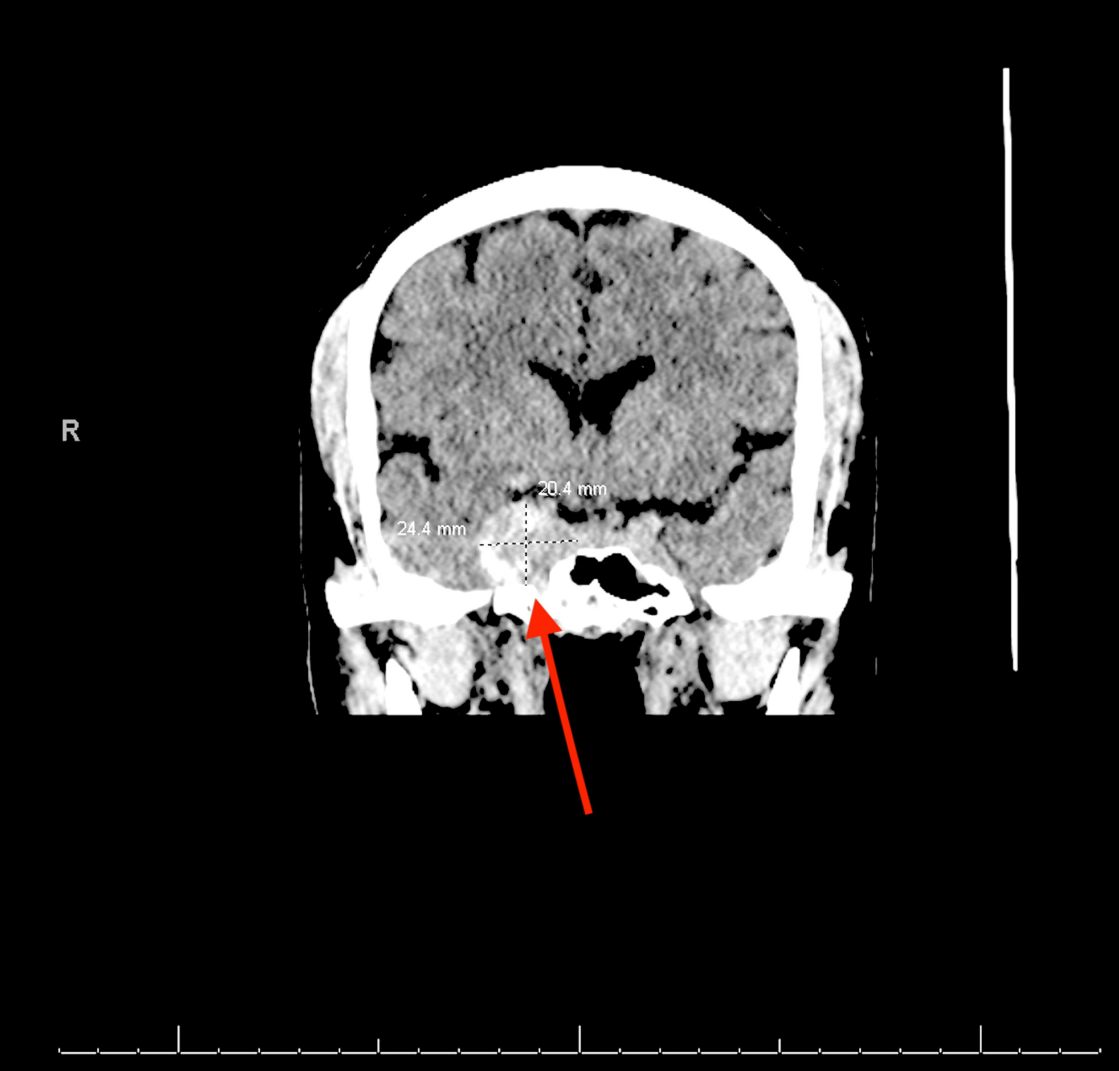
Original CT head from the emergency department The arrow points to the aneurysm within the cavernous sinus.
